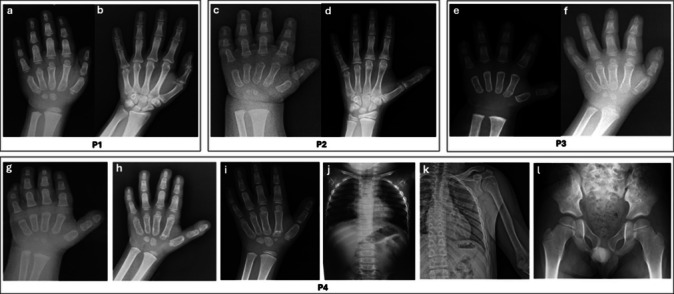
Hand radiographs of four patients (P1–P4) with geleophysic dysplasia (a–i), posteroanterior chest radiographs (j and k), and a pelvic radiograph (l) of P4. P1 had delayed carpal ossification, short fourth and fifth metacarpal bones, broad proximal phalanges, and short middle and distal phalanges at 7.6 years of age (a); these findings were less pronounced at 24.1 years (b). P2 exhibited very small carpal bones, short third–fifth metacarpal bones with rounded proximal ends, and short phalanges at 1.8 years (c); however, on the follow-up hand radiograph at 13.7 years (d), these findings were less pronounced. P3 had non-ossified carpal bones, short tubular bones, and broad proximal phalanges at 1.5 years (e); shortening of tubular bones and delayed bone age persisted at 5.1 years (f). In addition to short tubular bones and broad proximal phalanges, P4 showed shortening of the fifth middle phalanx at 0.2 (g), 4.7 (h), and 7.2 (i) years. P4 also had rib widening that became more pronounced with age, observed at 0.2 (j), and 16.5 (k) years; whereas the pelvic radiograph of P4 showed coxa valga and short femoral necks at 7.2 years of age (l)
